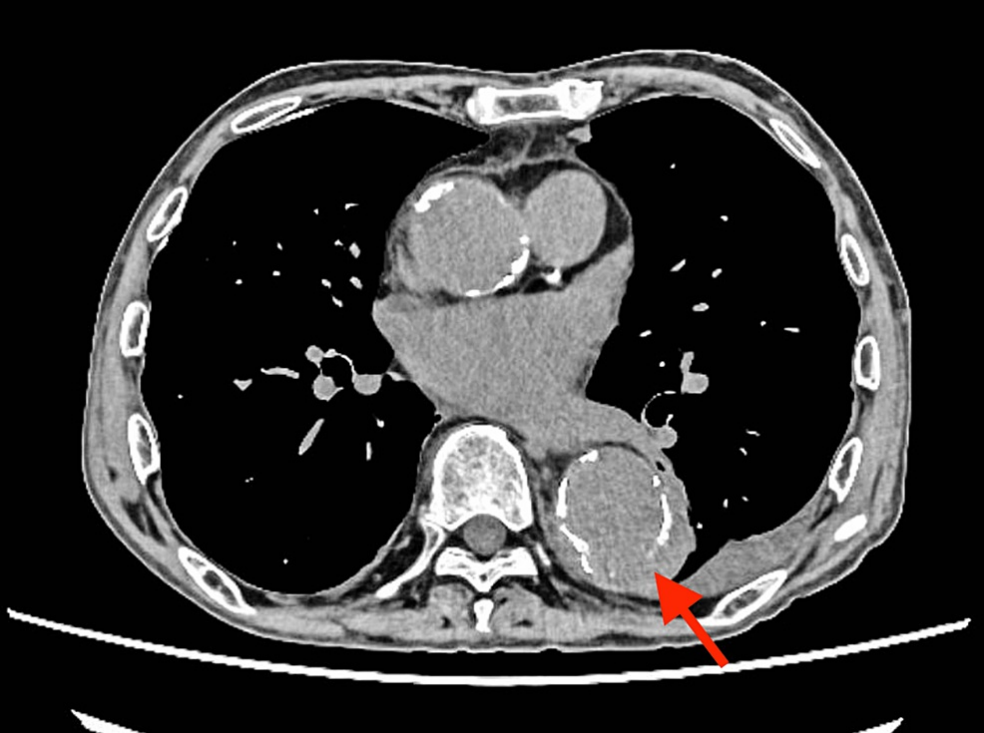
CT findings showing thoracic aorta with suspected partial vessel wall disruption (red arrow)
